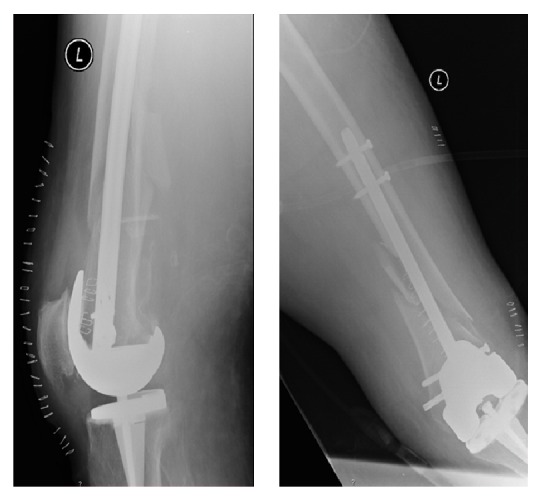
Immediate postoperative AP and lateral X-rays showing a left periprosthetic knee fracture, stabilized with retrograde intramedullary nailing technique.
